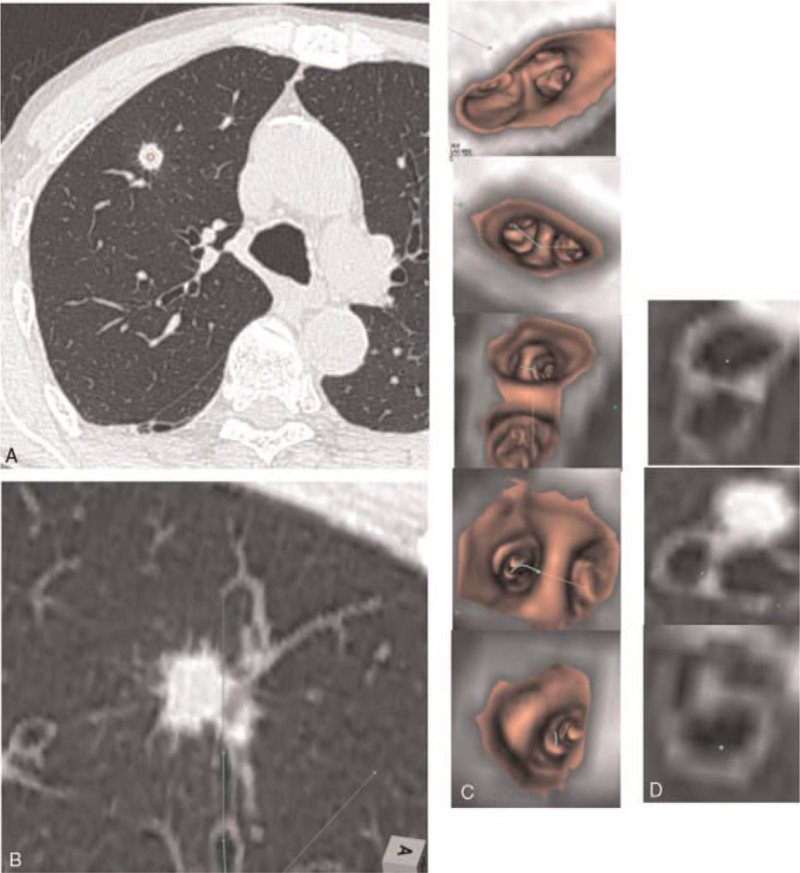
(A) No bronchus sign is seen using axial computed tomography (CT). (B) Image modification using the direct oblique method on CT images to draw the route to the target. (C) As for conventional virtual bronchoscopic (VB) navigation systems, VB images of each bronchial branching on the route to the lesion are shown as an animated image, and the bronchi for bronchoscope advancement are marked. (D) To confirm the peripheral bronchi from the limit of the VB image animation, the bronchial representation shifts to oblique CT, maintaining the visual axis seamlessly with fusion images created from the VB images and oblique CT images.
